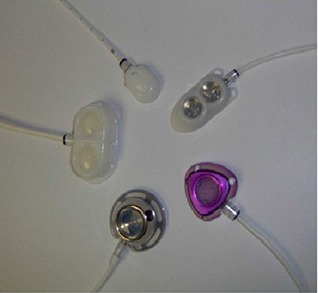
A sample selection of Bard Access ports used in this study. Starting at the 2 o'clock position, ports are identified as the Rosenblatt model 0654970, Titanium PowerPort model 1708000, Titanium Full Size port model 0605300, MRI Dual Lumen port model 0605930, and the Ultra Low Profile port model 0655640.
